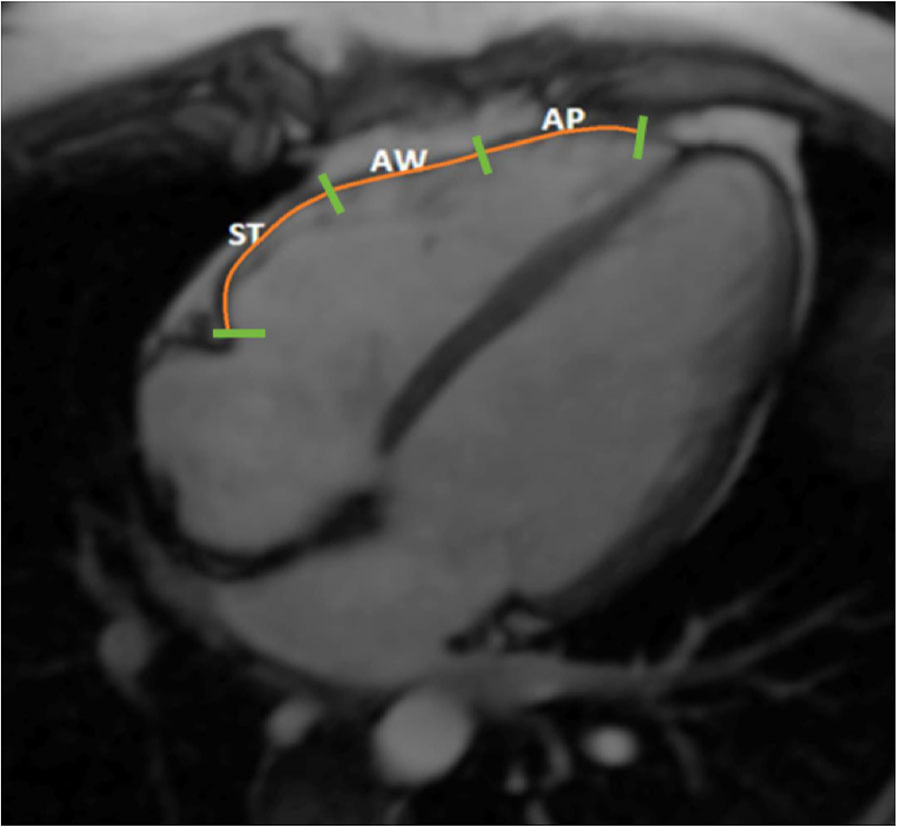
Representative right ventricular segmentation used in Feature Tracking Cardiac Magnetic Resonance Imaging. Abbreviations: ST = subtricuspid region; AW = anterior wall region; AP = apical region
